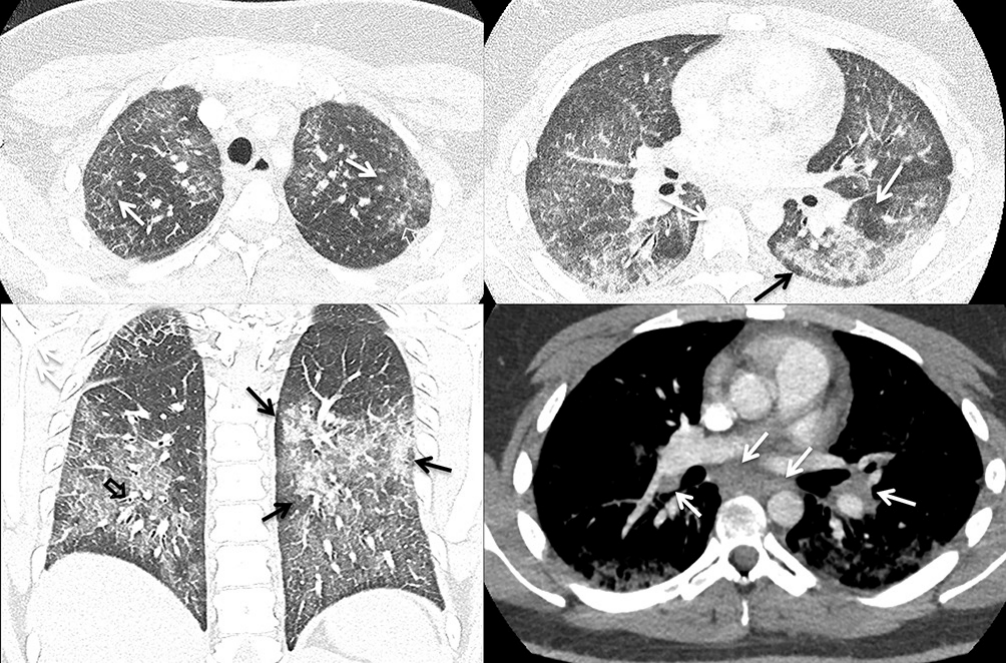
A (top left). Axial HRCT image showing both centrilobular (white arrows) and peribronchovascular (open white arrow) in the apices B (top right). Axial HRCT image showing peribronchovascular groundglass and consolidation with lobular (white arrows) and subpleural (black arrow) sparing C (bottom left). Coronal CT image showing peribronchovascular groundglass and consolidation (black arrows), bronchial wall thickening (black open arrow), and mild septal thickening (white arrows) D (bottom right). Axial CT image showing mild subcarinal and hilar lymphadenopathy (white arrows). HRCT, high-resolution CT.
